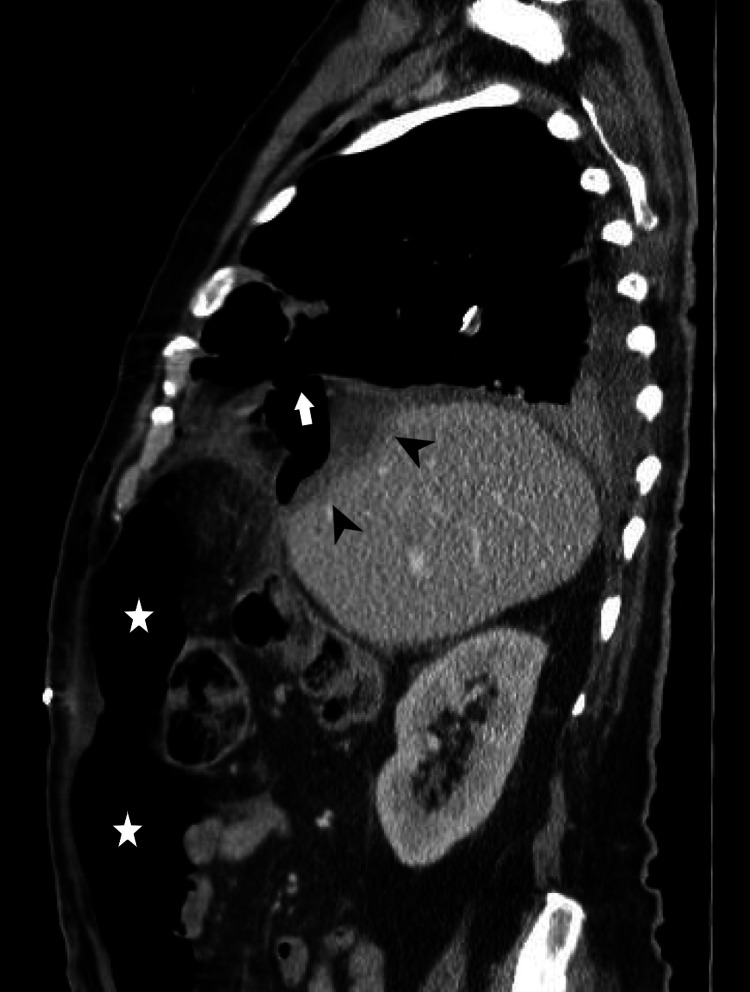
Sagittal CT scan of the patient's thorax and upper abdomen. The image displays a residual hepatic abscess following surgical drainage, indicated by the arrowheads. The arrow marks the diaphragmatic perforation, while the stars identify an extensive pneumoperitoneum.
